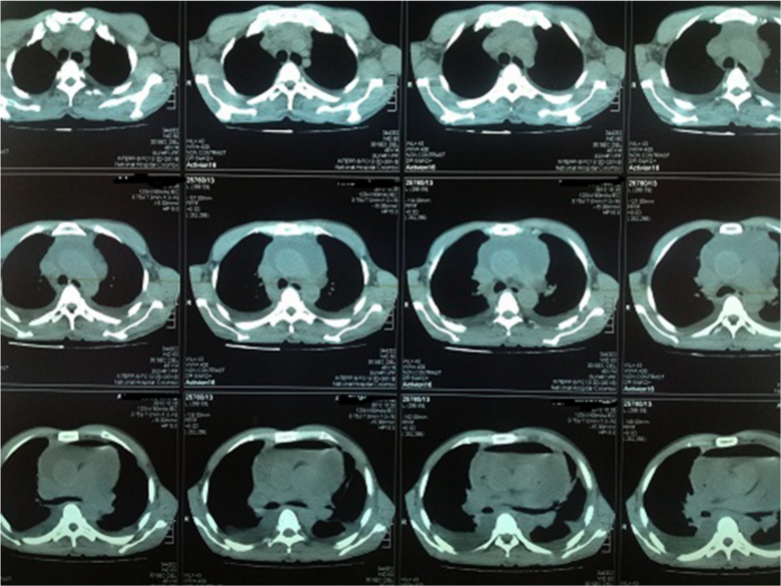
Contrast Computer Tomography Chest – Contrast Computed Tomography Chest showing air and fluid around the heart.
